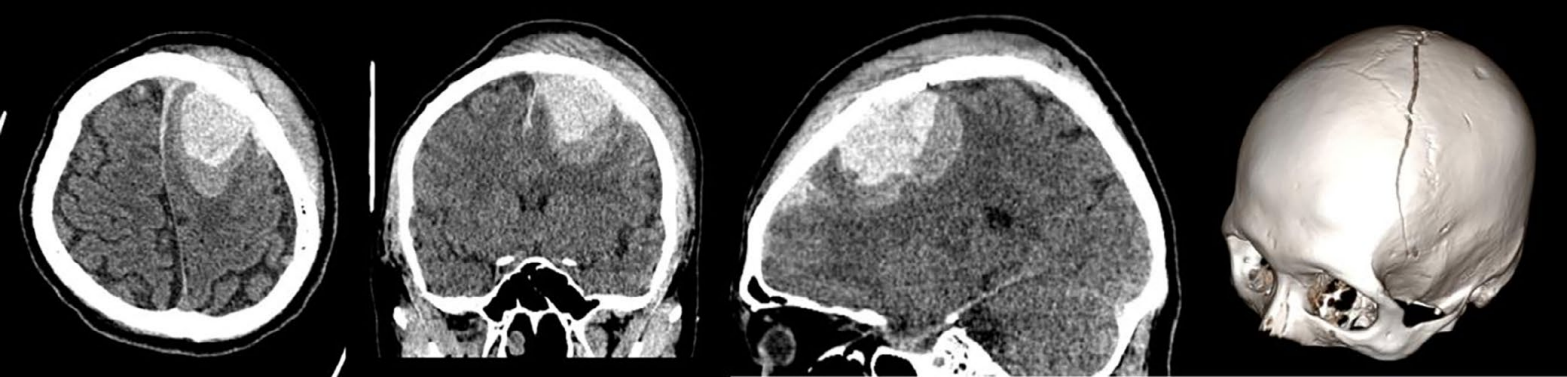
Computed tomography (CT) images showing skull fracture, intracerebral hematoma, subdural hemorrhage as well as multiple punctate hemorrhagic foci caused by TBI.
